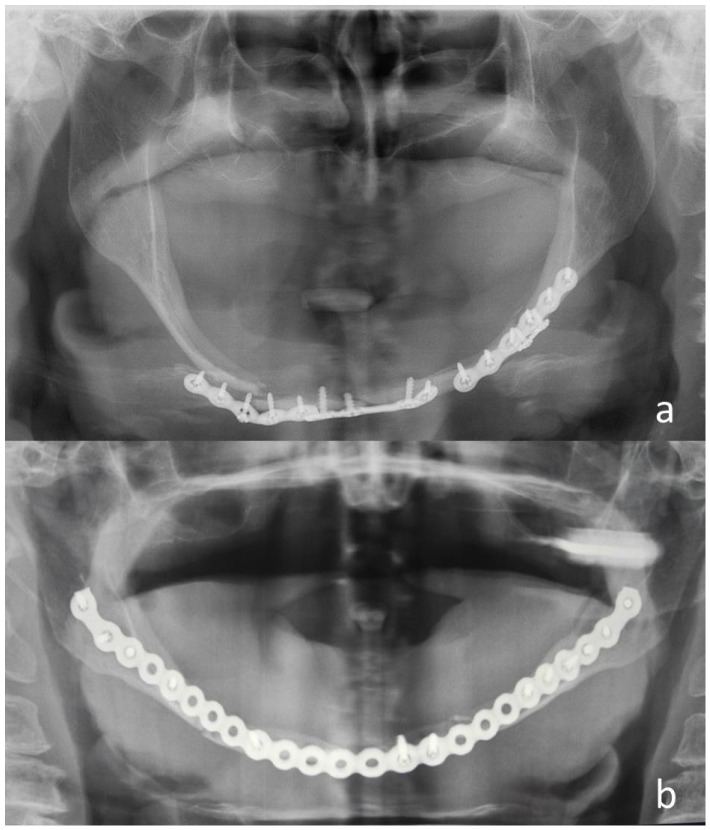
(a) Panoramic radiograph showing inadequate fixation in patient No. 18 with Stage III atrophy; (b) postoperative panoramic radiograph showing ORIF with a 2.0 mm titanium locking plate.
